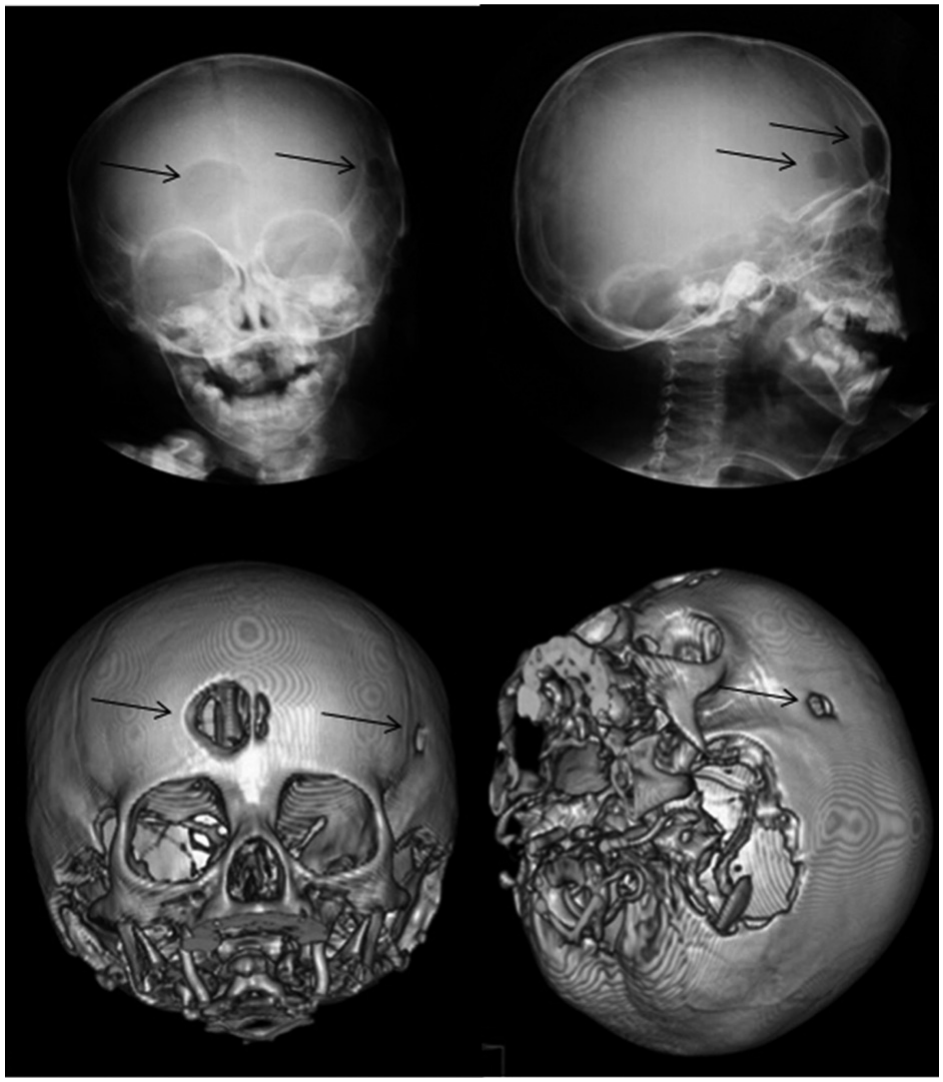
Frontal and lateral radiograph of the skull showing a punched-out lesion in the frontal and temporal bone (arrows). A 3-dimensional CAD view of cranial bones of the same patient demonstrating osteolytic lesions (arrows).
